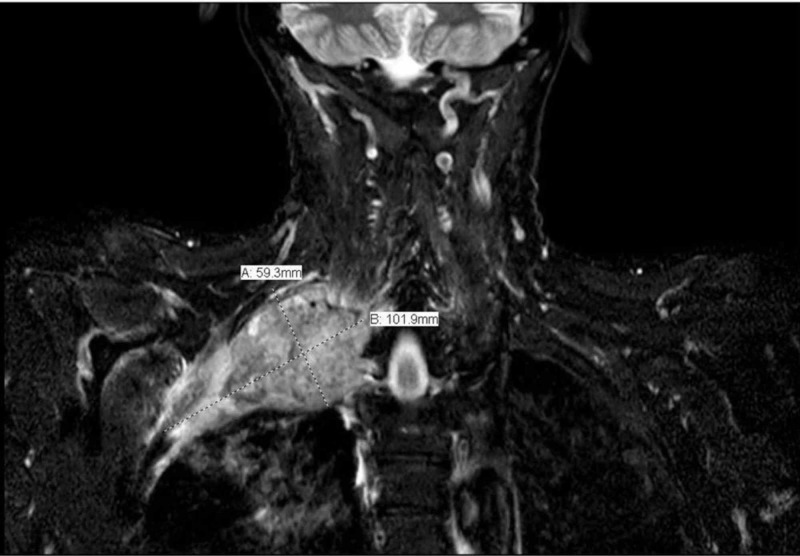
MRI of size, location and extent of right Pancoast tumor MRI, magnetic resonance imaging
